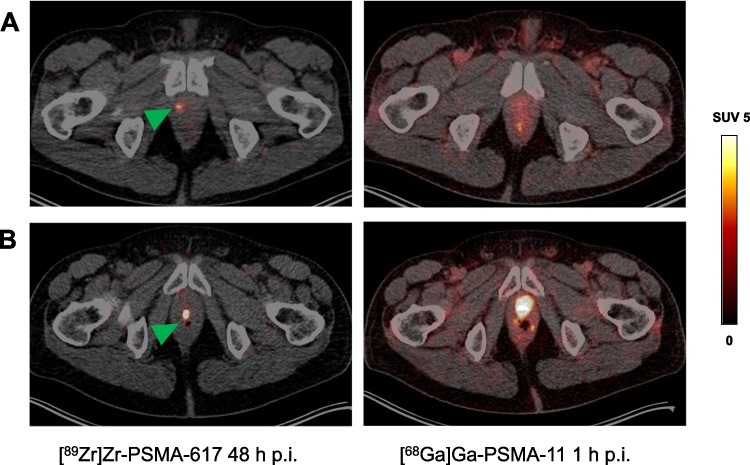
Transversal slice images showing presumed local recurrence of prostate cancer (green arrows) revealed by [^89^Zr]Zr-PSMA-617 PET/CT (left-hand column) but not identified on [^68^Ga]Ga-PSMA-11 PET/CT (right-hand column) in two patients (rows A and B, respectively). p.i., post-injection; SUV, standardized uptake value
